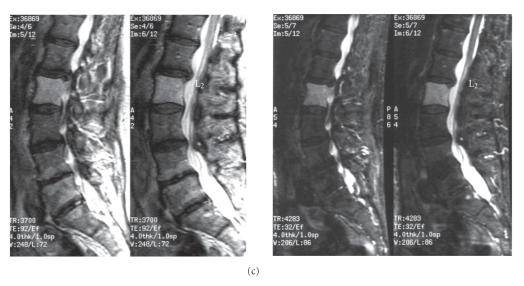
(a) The PET scan did not demonstrate increased glucose uptake in the lumbar spine. (b) and (c) Axial and sagittal MRI demonsrated abnormal signal intensity in the second lumber vertebrae.
